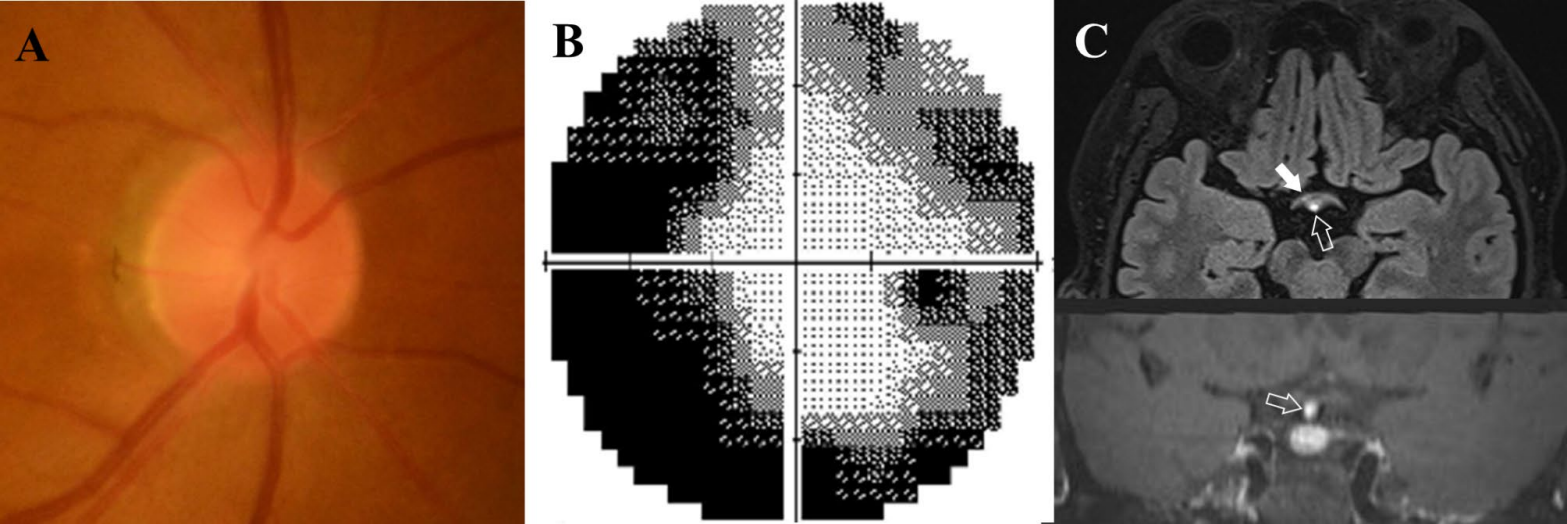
Images of the right eye at follow-up examination. After 10 months of corticosteroid treatment, despite the optic disc photograph of the right eye showing no change (A) compared with that at the initial exam, Humphrey 30−2 visual field shows generalized peripheral depression in the right eye (B). Follow-up orbital magnetic resonance imaging demonstrates newly developed focal enhancement near the junction of the right optic nerve and chiasm (C, upper panel, filled arrow) and enhancement of the pituitary stalk (C, upper panel, blank arrow; see higher magnification image with an arrow in the lower panel), suggestive of sequential sarcoidosis-associated optic neuropathy
